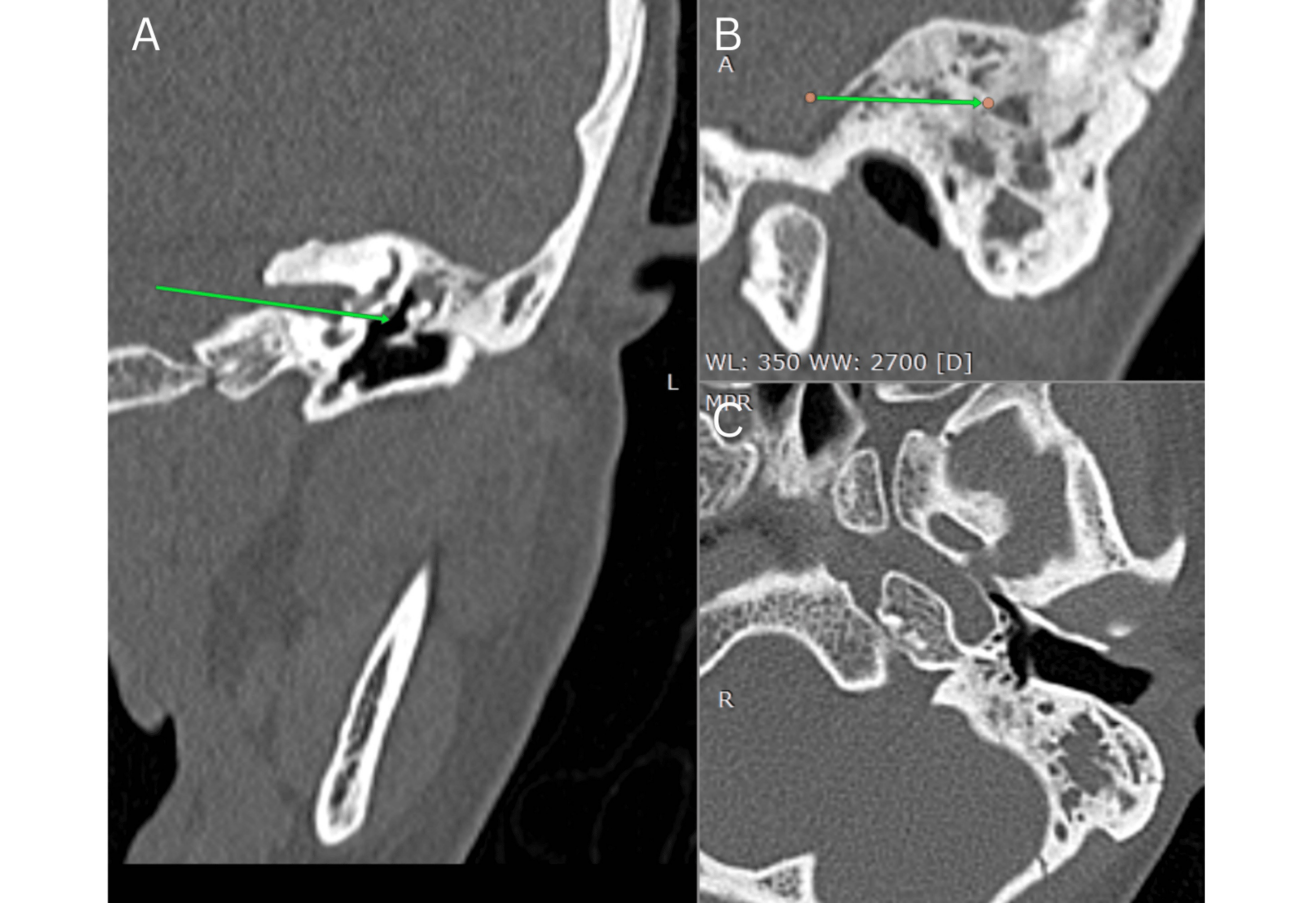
High-resolution computed tomography of the temporal bone (September 2025): near-complete resolution (A) Sagittal/oblique view: near-complete resolution of inflammatory changes in the middle ear and mastoid; green arrow highlights fully re-aerated cavities. (B) Axial view: mastoid air cells are fully re-aerated with well-delineated bony septa. (C) Coronal view: normal aeration of the middle ear and mastoid with intact bony margins; minimal residual mucosal thickening reflects prolonged radiologic cure.
